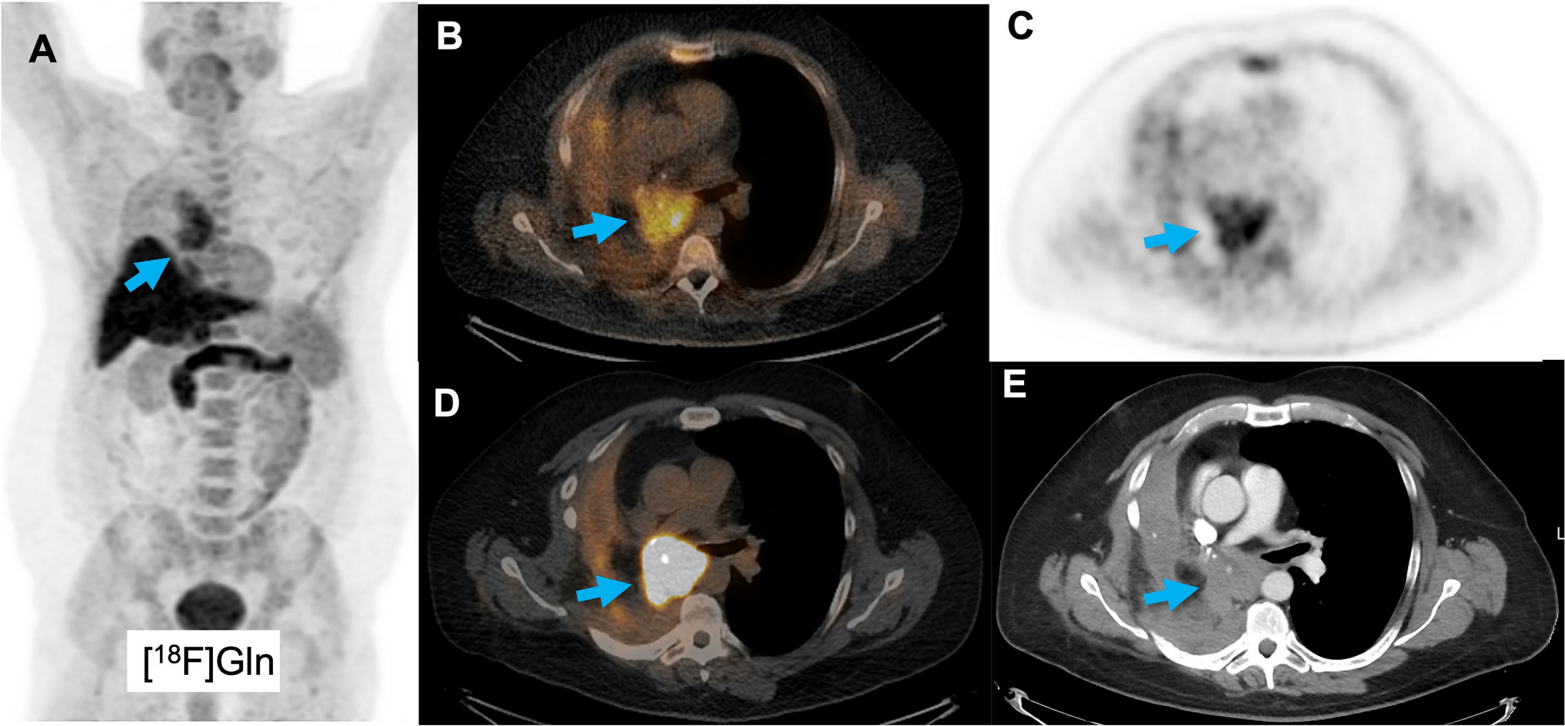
A patient with a squamous cell lung cancer lesion (arrow) scanned with [^18^F]Gln, showing increased uptake (A–C). Corresponding [^18^F]FDG images show increased uptake as well (D, E).
